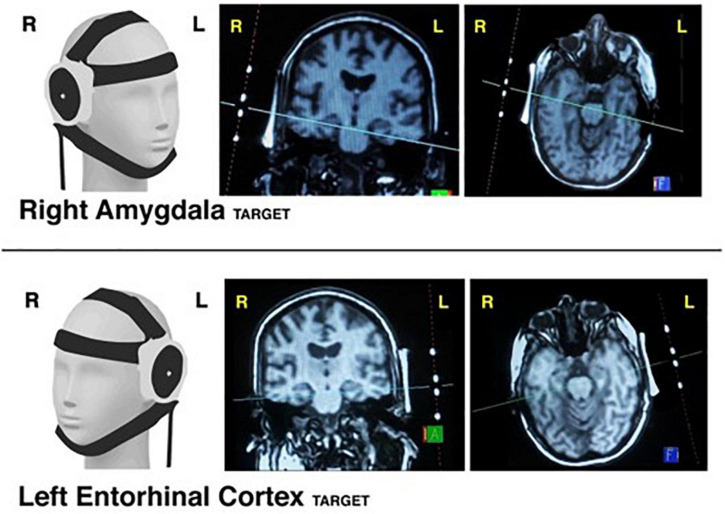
tFUS targeting. Visualization of transducer placement on 3D model (1^st^ column) when targeting the right amygdala (Top Row) and left entorhinal cortex (Bottom Row). Examples of MRI-console guided targeting using transducer fiducial markers are provided in coronal view (2^nd^ column) and axial view (3^rd^ column).
